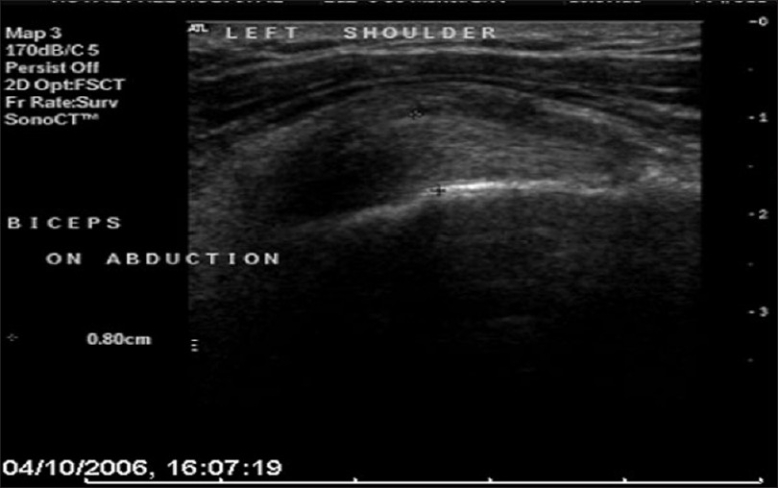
Ultrasound image of a longitudinal view of the LHB in abduction, demonstrating increased tendon diameter
